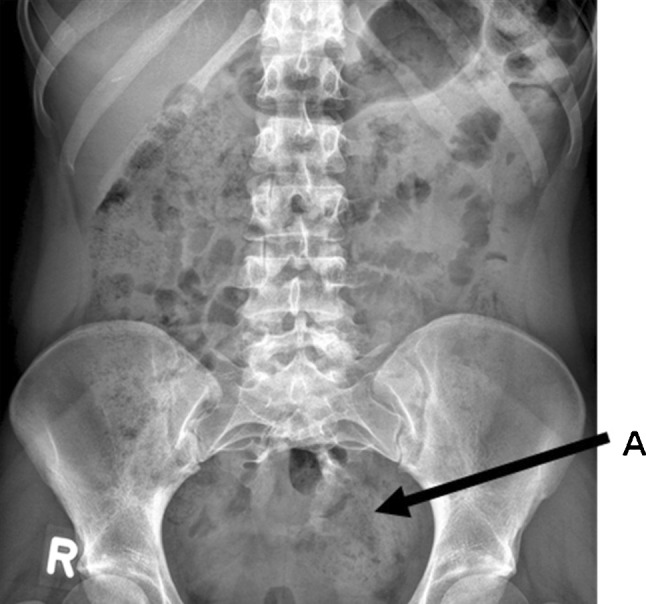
Pelvic X-ray *Arrow A*: the partial sacral agenesis
